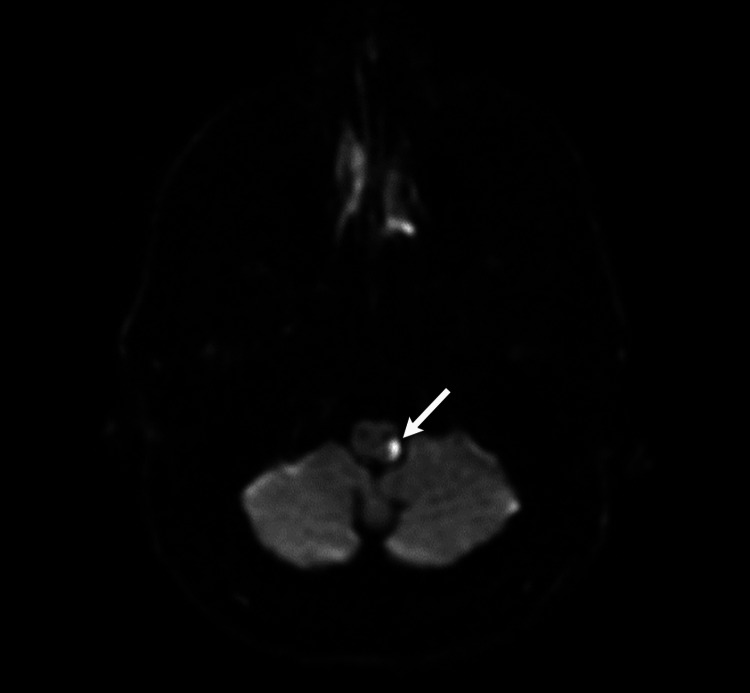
Diffusion-weighted MRI of the brain without contrast showing a small area of diffusion restriction in the left lateral medulla (arrow). Fast imaging employing steady-state acquisition and magnetic resonance angiography with contrast did not show any evidence of microvascular compression of the trigeminal nerve (not pictured).
